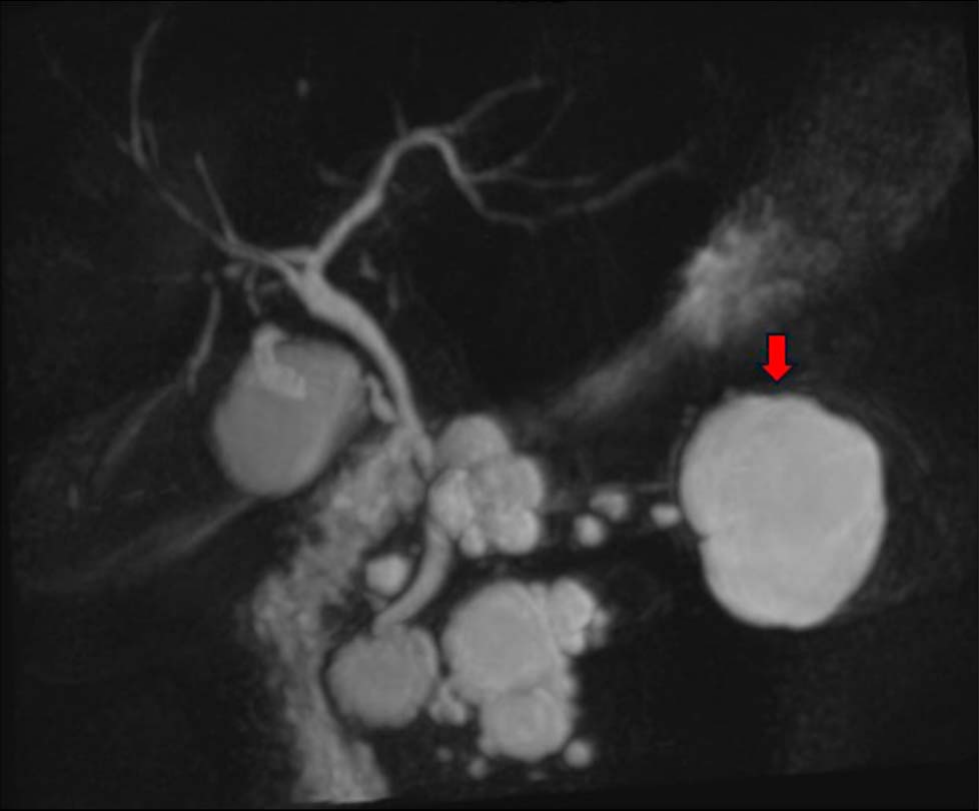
MRI/MRCP demonstrating multiple pancreas cysts. The arrow indicates the largest cyst in the distal pancreatic body that was targeted for biopsy in endoscopic ultrasound.
